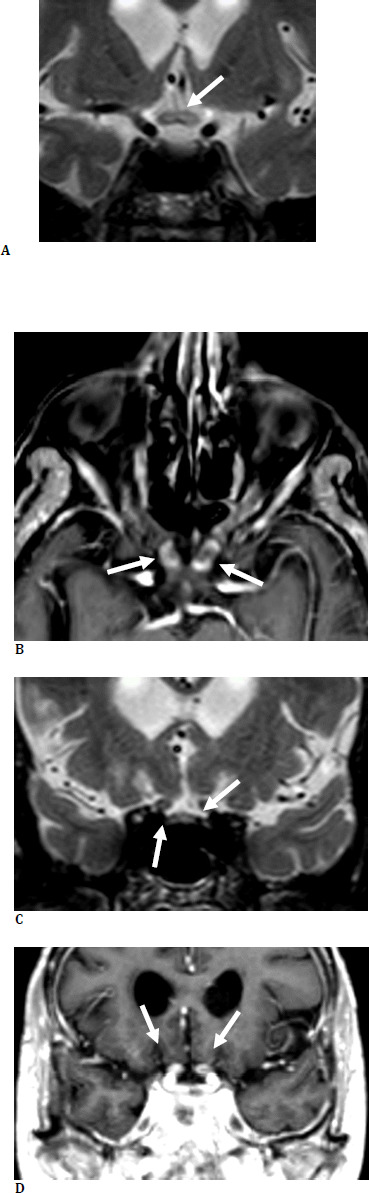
Brain MRI: Axial and coronal sequences showing thickening and STIR hyperintensity of the optic chiasm (A) and prechiasmatic segments of both optic nerves (C) (arrows), associated with contrast enhancement T1-weighted images (B, D) (arrows)
